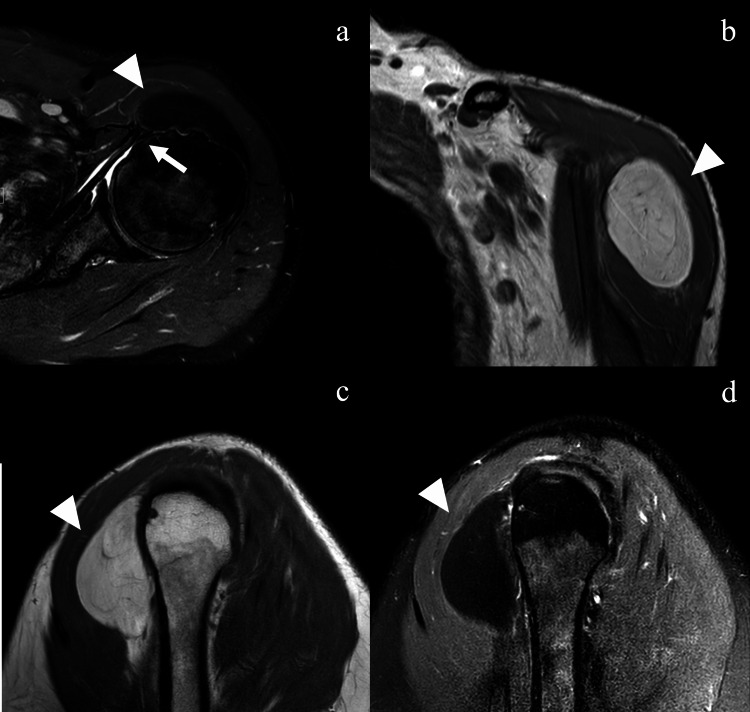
Preoperative magnetic resonance imaging Preoperative axial MRI show mass obliterated anterior aspect of lesser tuberosity (a, arrowhead) with full thickness tear of the subscapularis tendon and subluxation of the long head biceps tendon (a, arrow). The oblique coronal and sagittal MRI show a 46x35x48 mm homogeneous tumor under the deltoid muscle (b and c, arrowhead). The tumor appears signal-hyperintense on T1-weighted images (a and c) and suppressed on T2- weighted fat-suppression images (d).
